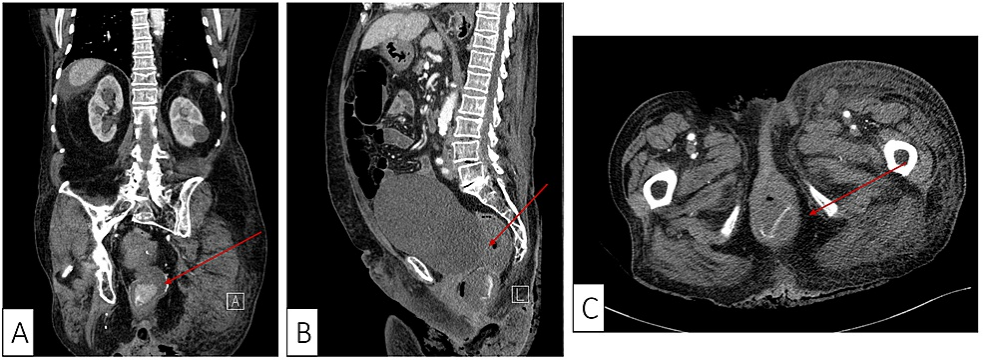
Contrast-enhanced computed tomography sections (A – coronal, B – sagittal, C – transverse) showing active contrast solution leakage in the rectal cavity (red arrow).
